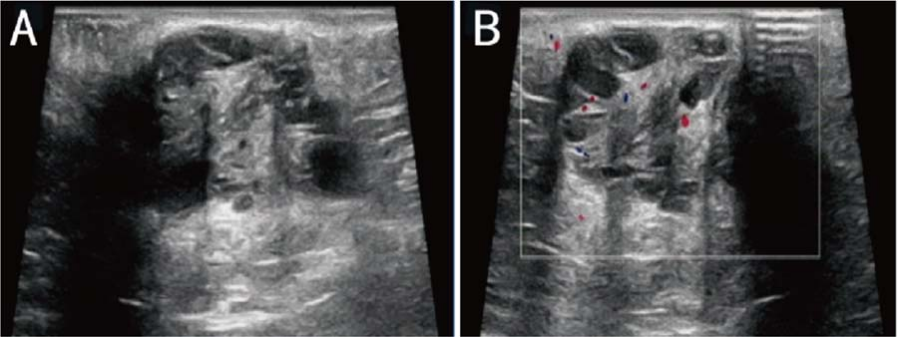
Ultrasound: (A) 2D ultrasound shows a mixed echo with a range of about 4 cm in the subcutaneous soft tissue around the left side of the anus, presenting as cystic-solid; (B) color doppler shows a small amount of punctate blood flow signal inside and around the mixed echo.
